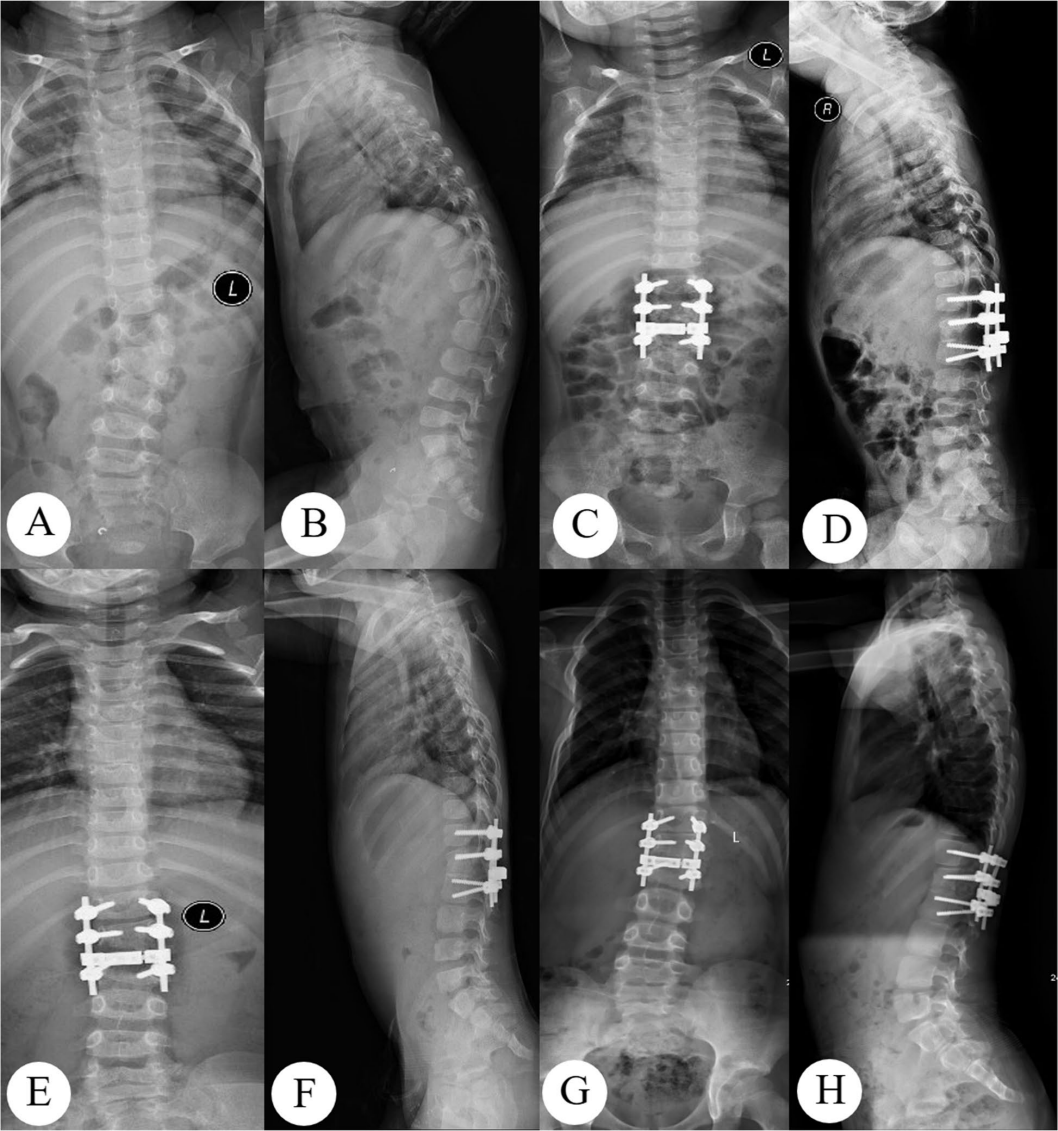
The female patient, age 1.5 years, underwent one stage posterior hemivertebrae resection and orthosis fusion. A, B Preoperative anteroposterior and lateral radiographs; C, D Postoperative anteroposterior and lateral radiographs; E, F Anteroposterior and lateral radiographs 15 months; G, H Last follow-up anteroposterior and lateral radiographs
